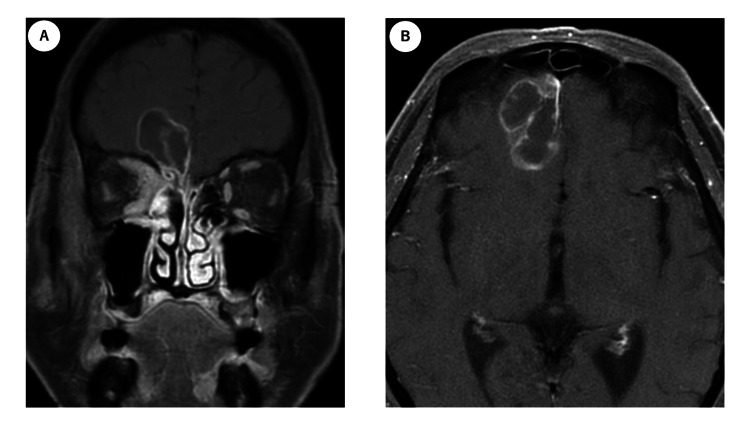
Post-treatment MRI after two months A, B: coronal and axial image The tumor of the paranasal cavity, shown in a T1-weighted image with gadolinium contrast enhancement, was significantly decreased. No noticeable changes were observed in the intracranial lesions.
